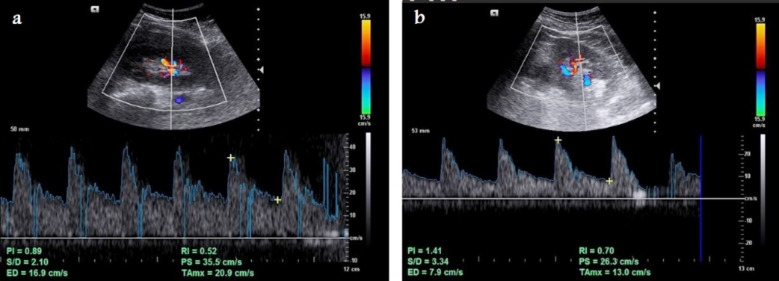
Selected spectral Doppler ultrasound images show Doppler RI of a) normal 52-year-old female individual with normal low resistance waveforms with RI=0.52, and b) diabetic 55-year-old female patient with RI=0.70 that is higher than normal.
